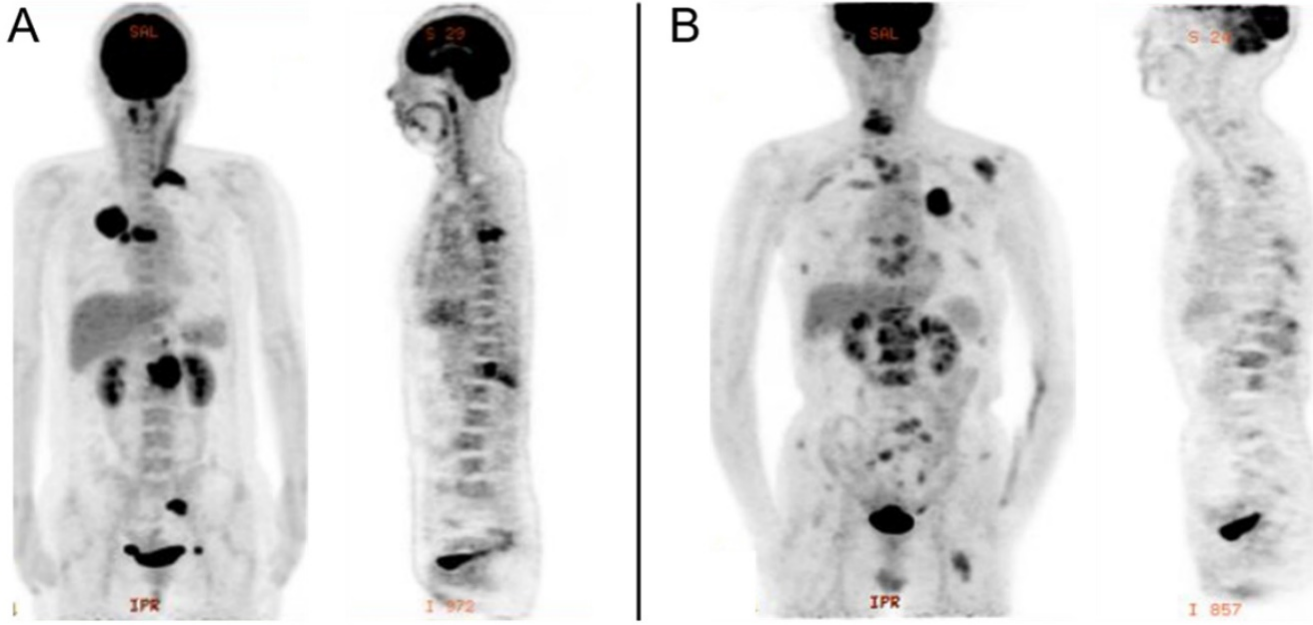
PET image of stage IV lung adenocarcinoma with bone metastasis. A, EGFR-wild: primary tumor in the right upper lobe (SUVmax, 20.3), with regional node (SUVmax, 11.4), and bone metastasis (SUVmax, 16.0). B, EGFR-mutant: primary tumor in the left upper lobe (SUVmax, 9.6), with intrapulmonary (SUVmax, 2.6), distant node (SUVmax, 2.4), adrenal gland (SUVmax, 6.1), and bone metastasis (SUVmax, 6.0).
